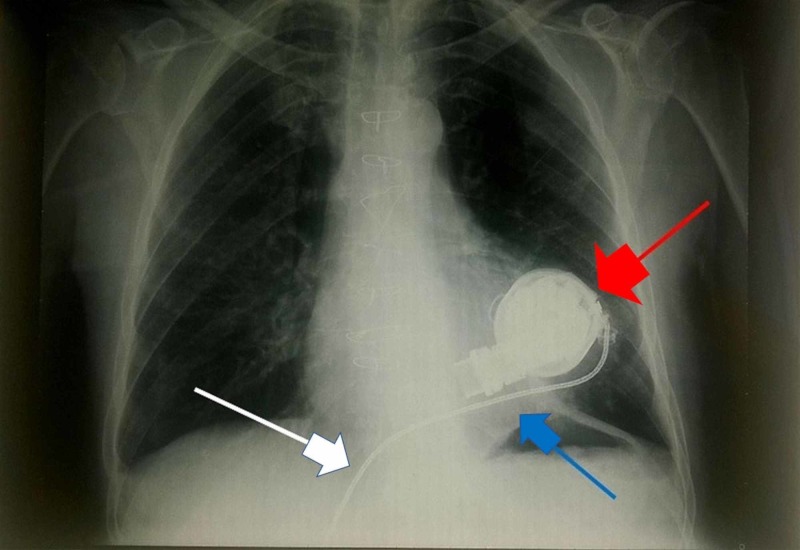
X-ray showing the position of the L-VAD The red arrow indicates the mechanical pump, the blue arrow indicates the left ventricle, and the white arrow highlights the driveline (an electric wire that connects the batteries to the pump).
